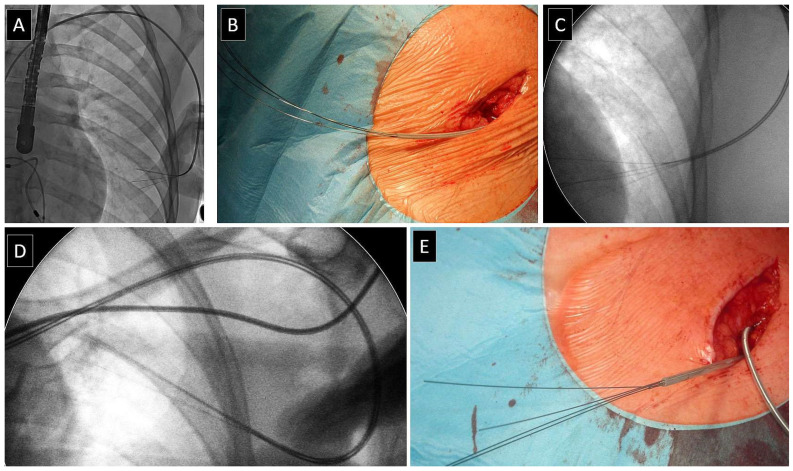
Construction of the older models of VDD and its impact on technique TLE. Older models of VDD leads have four independent helical conductors allowing the insertion of stylets (A–E). Stylet stiffen the lead by creating a splint (rail) for the dissecting catheter and reduce the risk of slipping off the ring electrodes. Sometimes the angulation of the leads makes it impossible to reach the end of the conductor with the stylet (A). The trick of introducing 4 styles is shown in 3 radiographs of 3 patients (A,C,D) and photographs of the surgical field (B,E).
